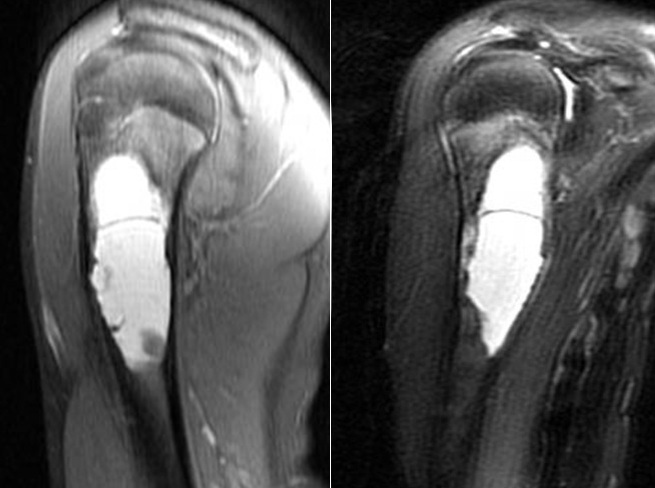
T2-weighted sagittal MRI depicting of the lesion
